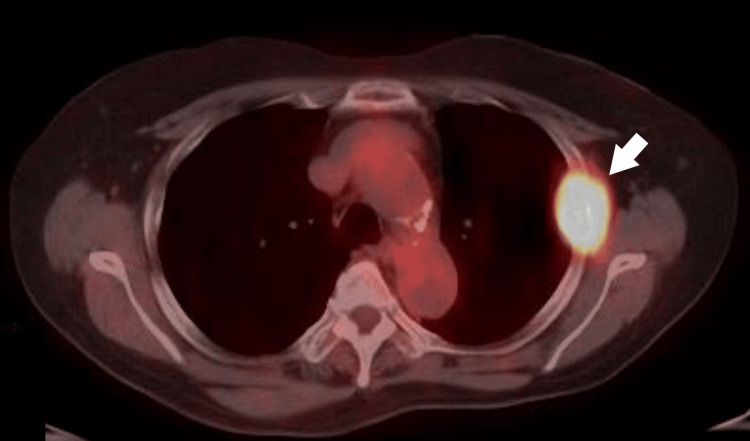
Fluorodeoxyglucose (FDG)-PET/CT image showing uptake in the rib lesion (SUVmax: 15.16, arrow).
